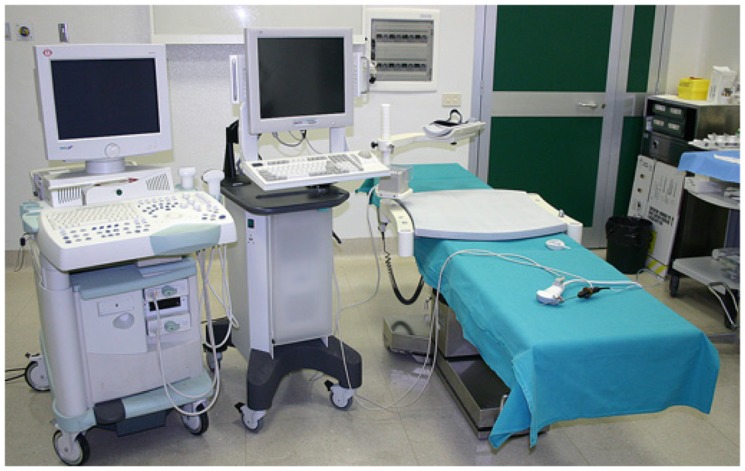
Image of navigator system: From left to right: Ultrasound machine, TECHNOS MPX, NAVIGATOR SYSTEM (with touch screen monitor) connected with the “navigation system”, composed by a passive electro-mechanical sensor (near to the patient) and the “active” one (attached to the ultrasound probe).
